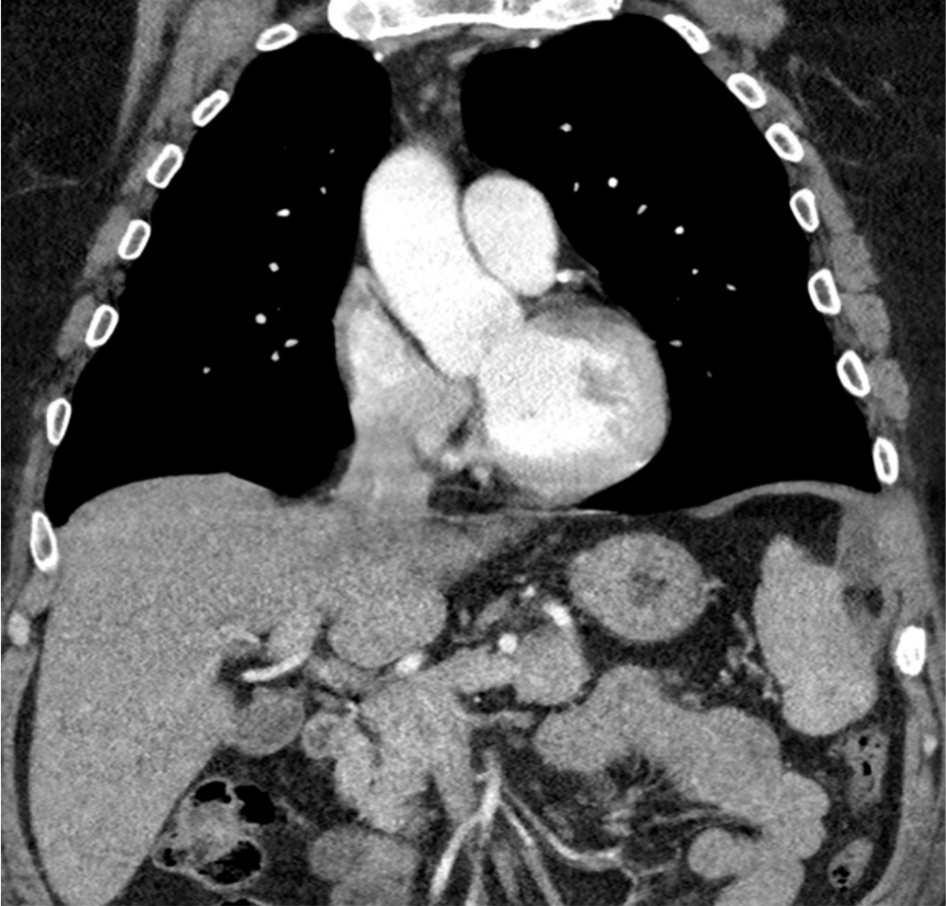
Postoperative CT scan showing reduced hernia with an intact diaphragm.
